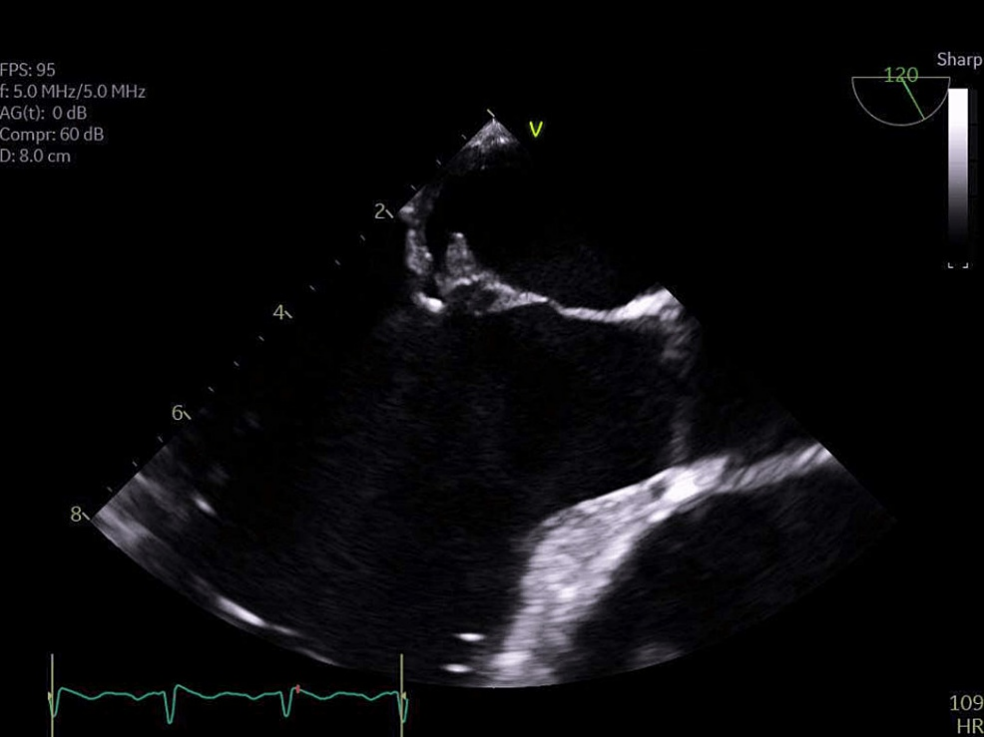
TEE image showing vegetations on the native mitral valve TEE, transesophageal echocardiogram
